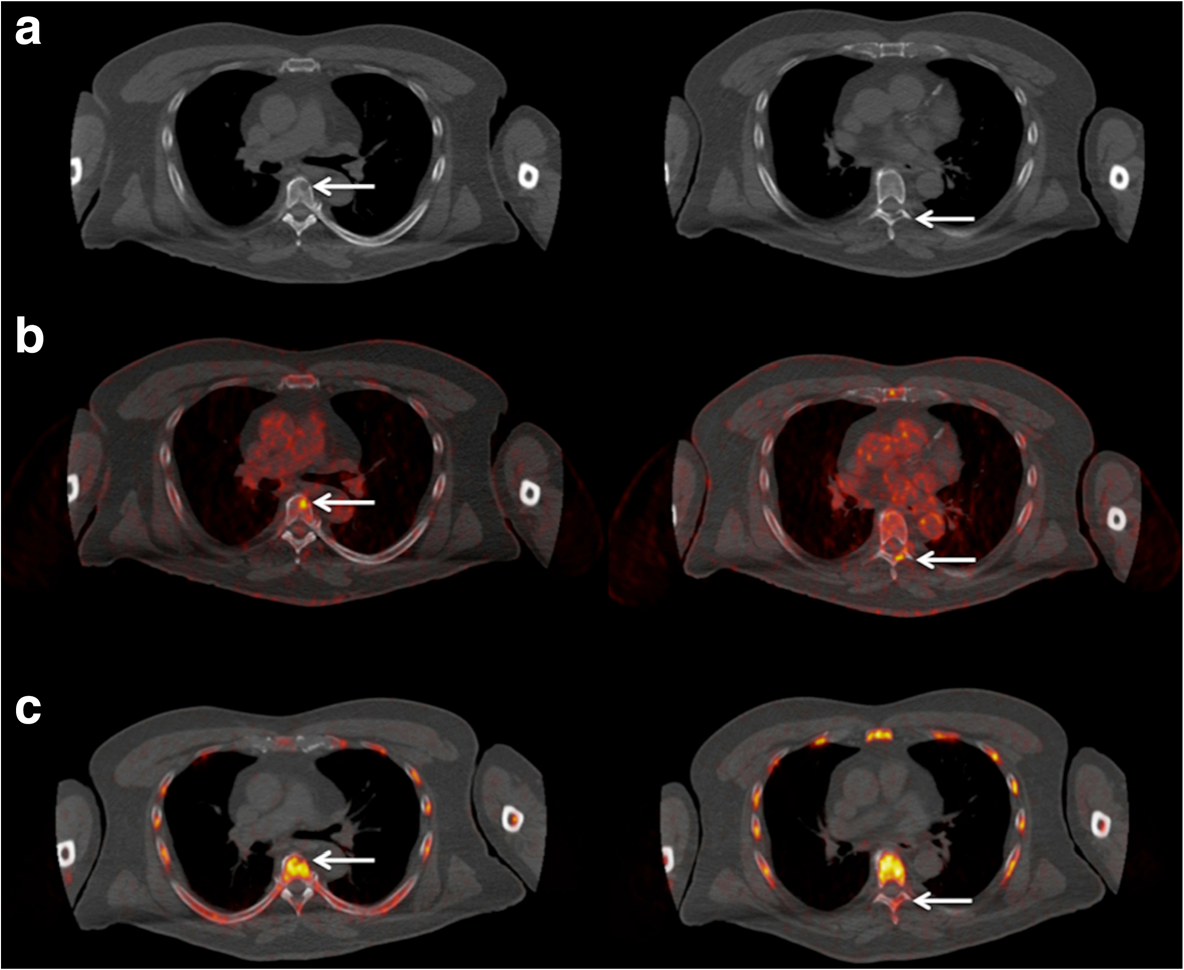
Transaxial low-dose CT (upper row, a), ^18^F-FDG PET/CT (middle row, b), and ^18^F-FLT PET/CT (lower row, c) at the thoracic level of a 65-year-old male patient with initial diagnosis of MM. Intense ^18^F-FDG accumulation (b) in osteolytic areas in the sixth thoracic vertebra (left column, a) and seventh thoracic vertebra (right column, a), reflecting active MM lesions. In contrary, ^18^F-FLT PET/CT revealed reduced tracer accumulation in the MM lesions (c), lower than the normal bone marrow uptake
